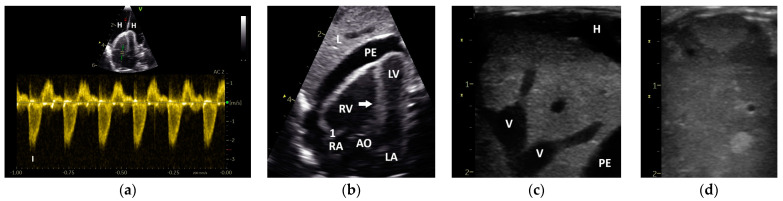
Sonographic findings of Congo grey parrots: (a) Insufficiency of the right atrioventricular valve (AV_right_) due to an enlarged right ventricle, with PW Doppler sonographic diastolic blood flow profile of the AV_right_ with a systolic insufficiency jet (I) with a very short acceleration phase; H: hepatoperitoneal cavity effusion. (b) End-diastolic movement of the septum in the direction of the left ventricle (arrow), as seen with B-Mode echocardiography; PE: pericardial effusion; RV: right ventricle; LV: left ventricle; RA: right atrium; LA: left atrium; AO: aorta; L: liver; 1: AV_right_. (c) B-Mode sonography of a congested liver associated with right heart failure, with compacted liver tissue and highly congested liver veins (V); H: hepatoperitoneal cavity effusion; PE: pericardial effusion. (d) B-Mode echocardiography of a liver with nodular liver tissue associated with the shape of the pulmonary blood flow profile, which had a short acceleration phase (outlier group 1).
